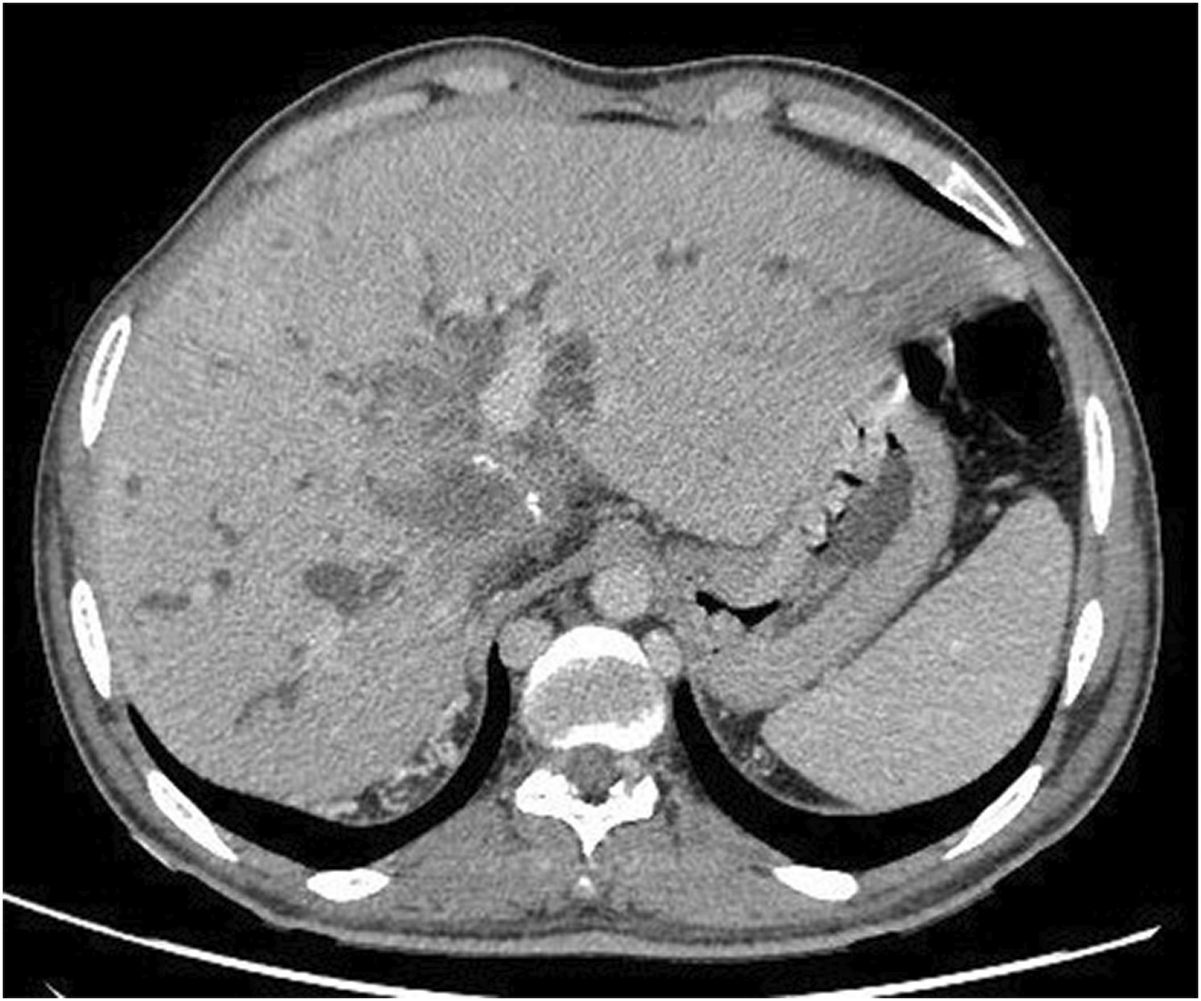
Axial abdominal CT SCAN demonstrating a low attenuating mass lesion seen in segment IV. There is a rim of fluid collection and fat stranding measuring approximately centimeters. Also, the lesion communicated with the common hepatic duct and left hepatic biliary tree, consistent with a calcified hydatid cyst.
